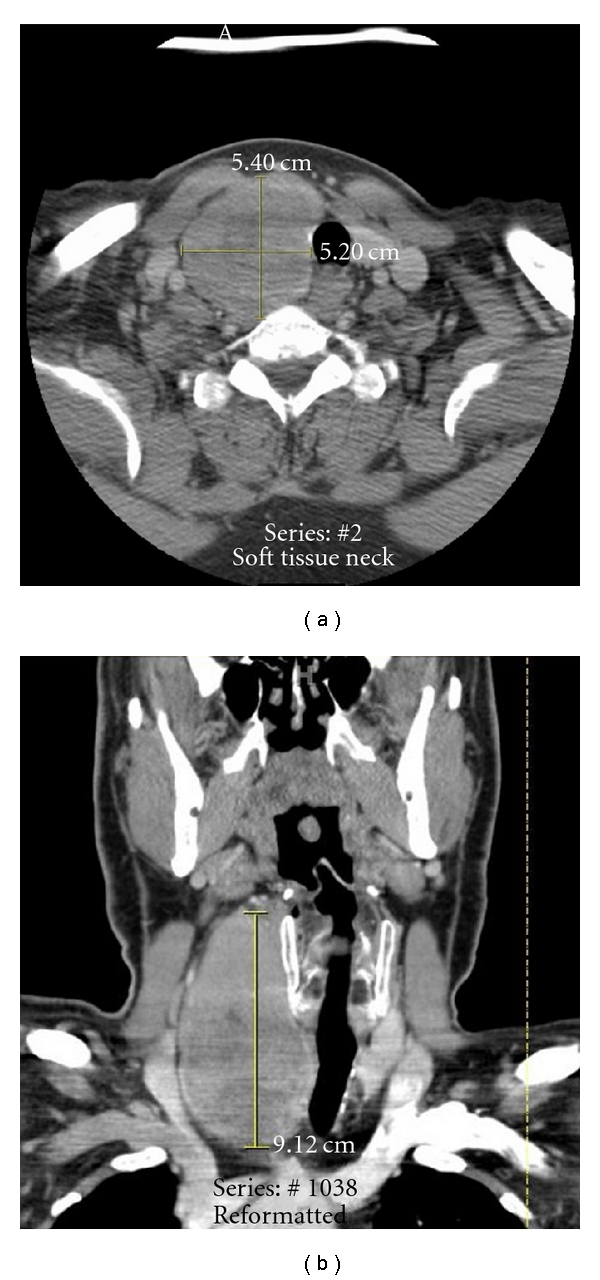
Preoperative computed tomography (CT) of the anaplastic thyroid carcinoma. (a) Axial view with contrast revealing a heterogeneous mass that is intimately associated with the trachea and pushes it toward the left side of the neck but free of the common carotid artery and the esophagus. (b) Coronal view with contrast demonstrating the craniocaudal extent and intimate tracheal involvement of the tumor.
